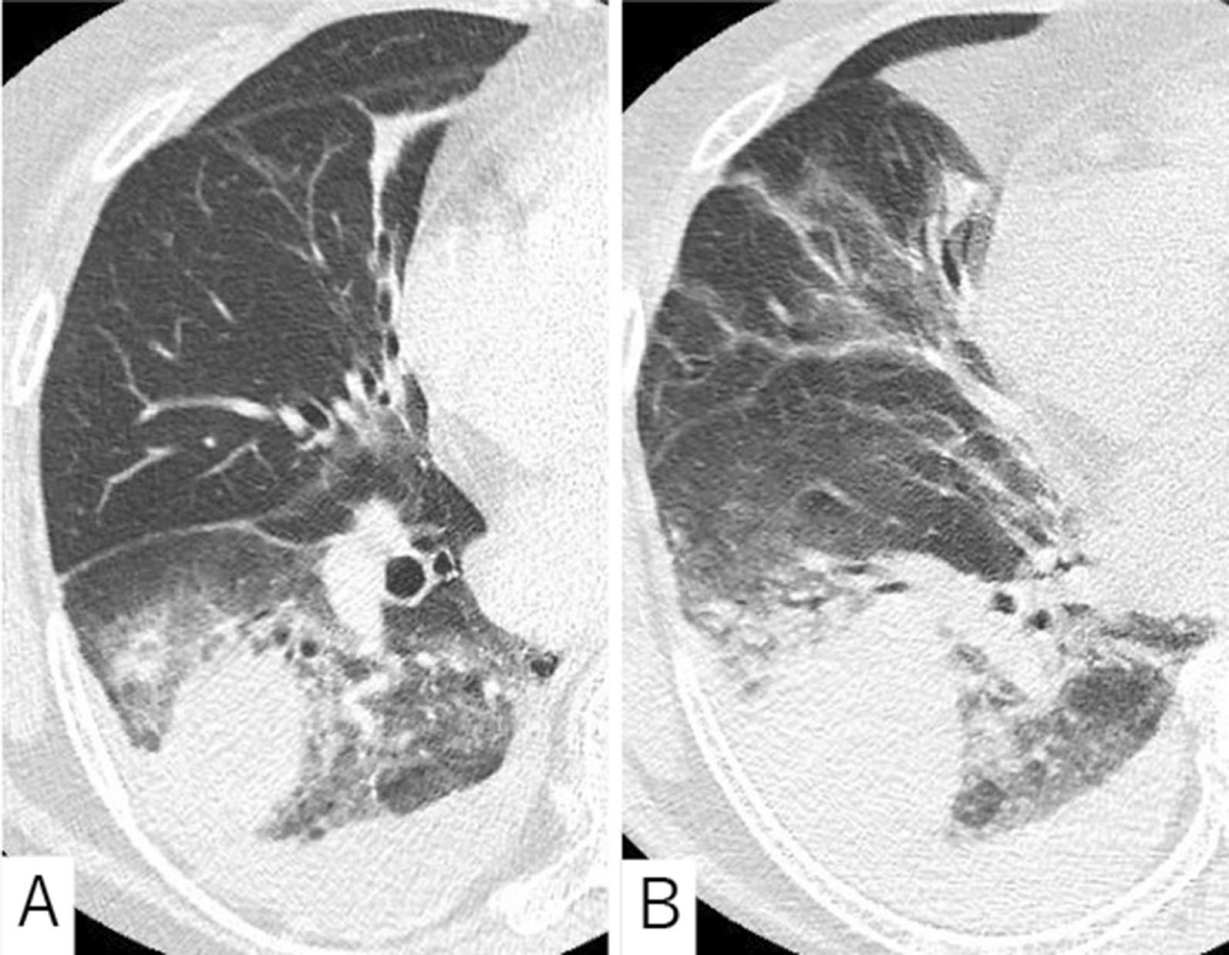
A A chest CT on arrival showing a mass with surrounding opacity in the right lower lobe with a pleural effusion. B A chest CT 7 h later showing a worsening pulmonary opacity and a pleural effusion
